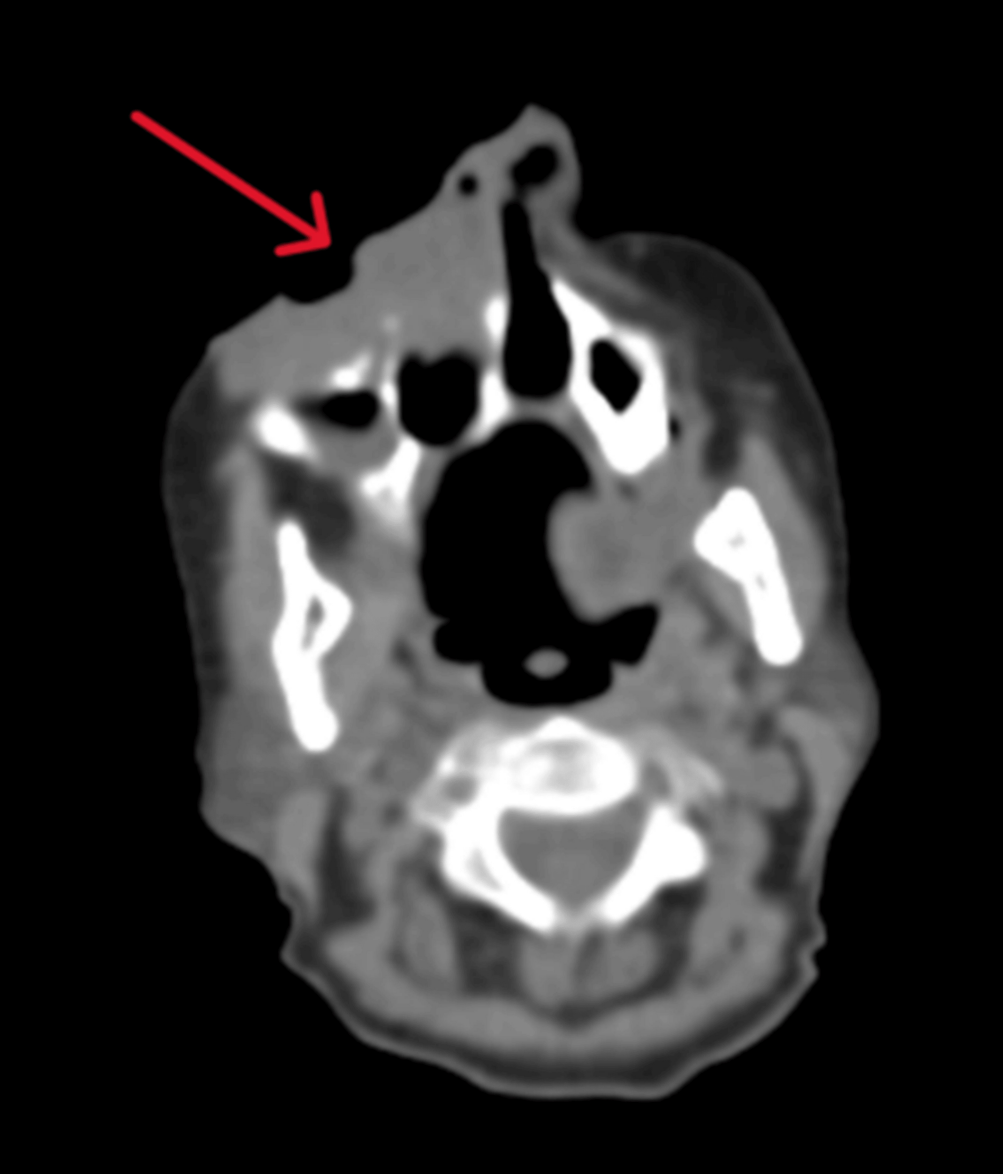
Axial CT scan of the head and neck showing extensive right facial basal cell carcinoma.
